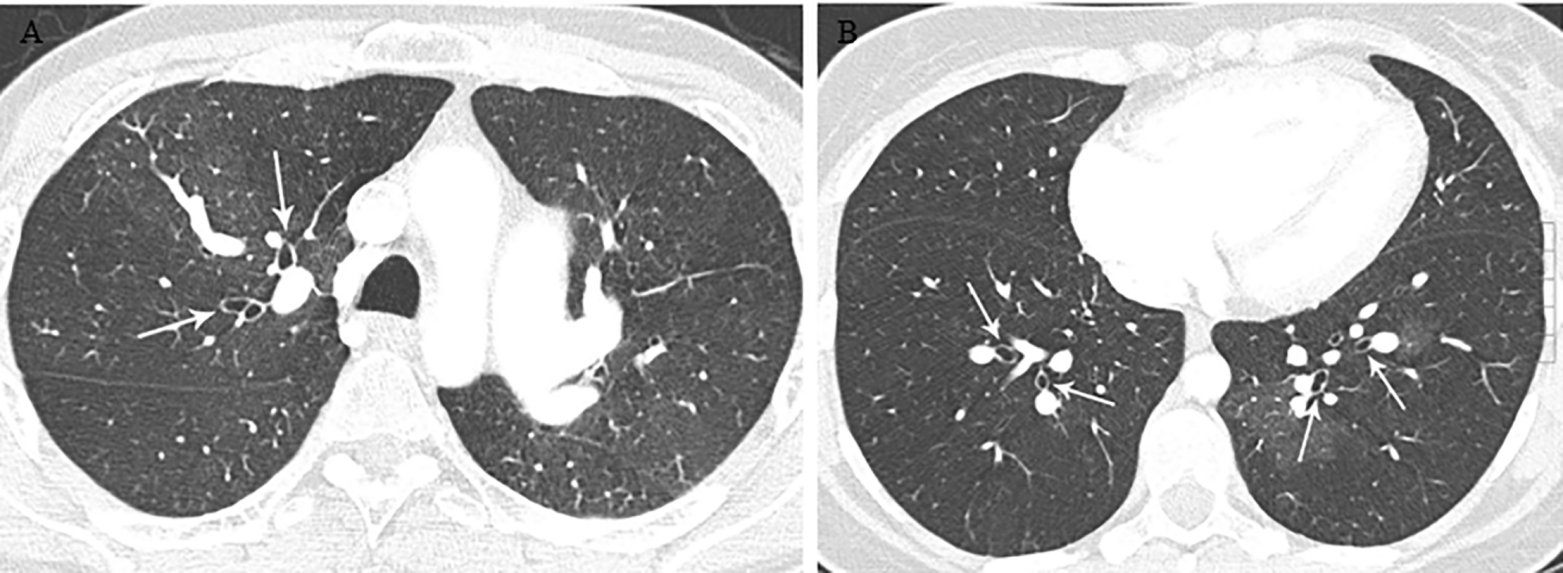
Bronchial dilatation in patients with CTEPH. (A) Axial CT image shows dilated bronchi (arrows) in the right upper lobe. (B) Axial CT image shows dilated bronchi (arrows) in both lower lobes.
